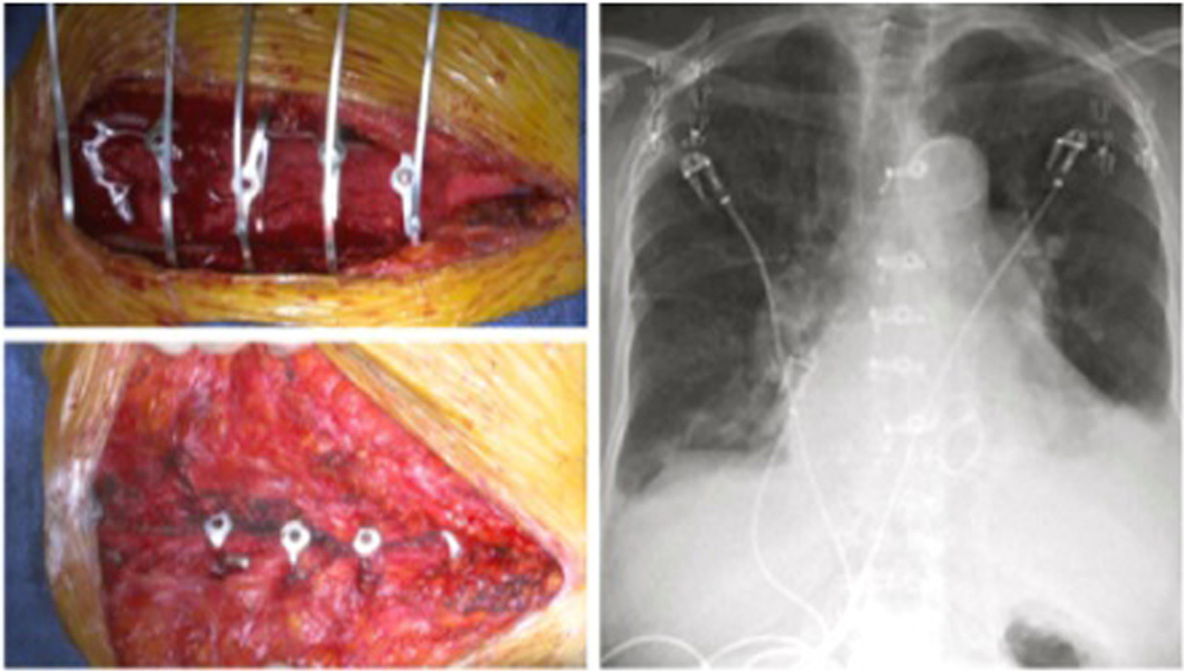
Intra-operative placement and post-operative X-rays. FlatWires are trimmed after all are placed, allowing for adjustments to be made. X-rays at day of discharge and 30 days confirmed alignment and proper placement of sternal constructs.
